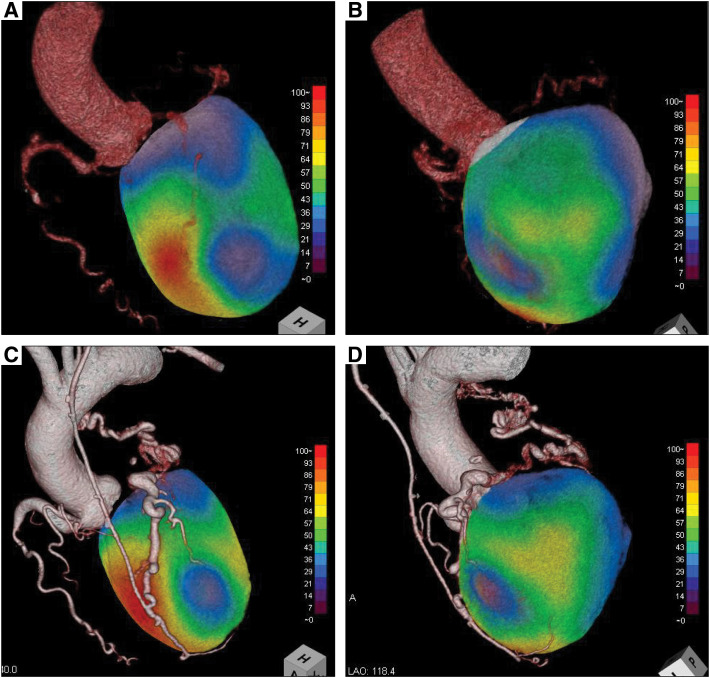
Technetium 99m sestamibi stress-rest myocardial perfusion scintigraphy. Exercise stress myocardial scintigraphy revealed infarction of the posterior inferior wall and severe ischemia of the lateral wall of the left ventricle (A and B). After the left internal thoracic artery-to-left anterior descending artery bypass, the myocardial ischemia from the lateral wall to the posterior wall of the left ventricle improved (C and D).
